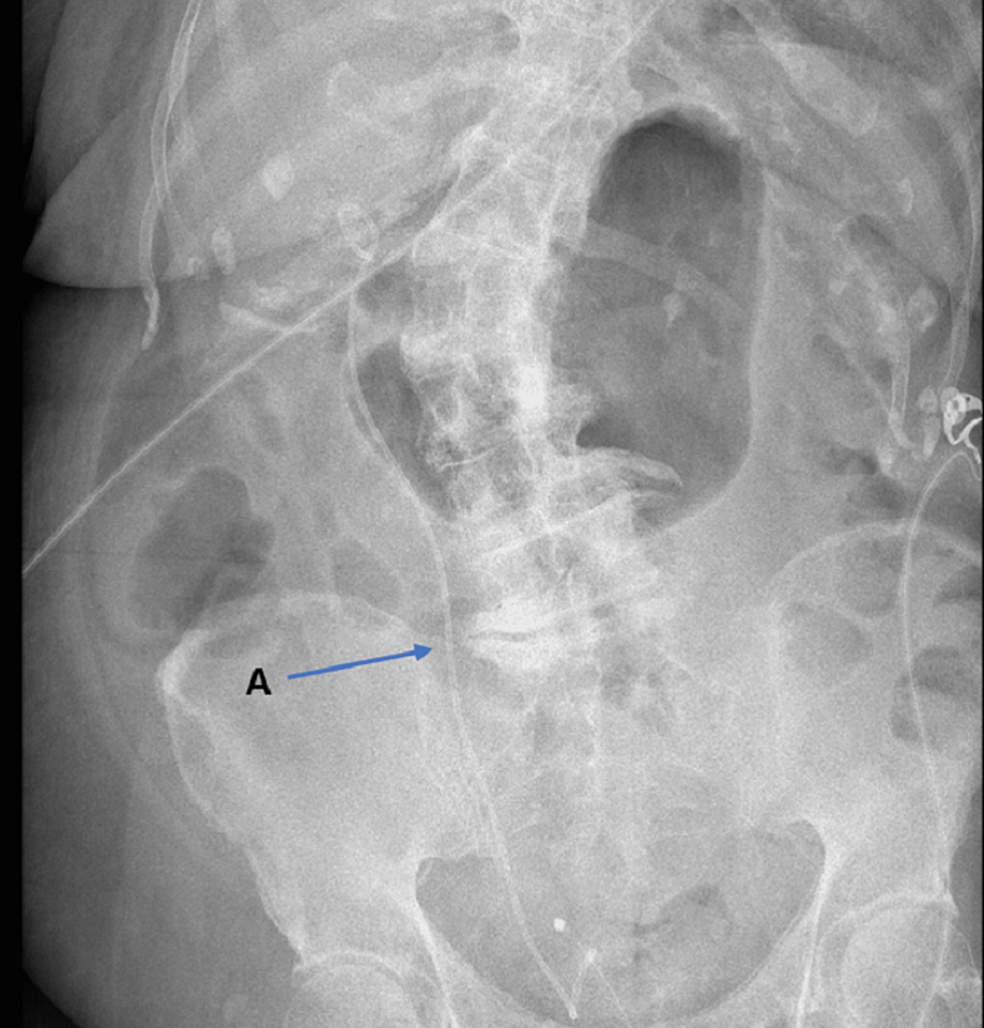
Satisfactory placement of a right-sided ureteric stent (A) on an abdominal x-ray.
